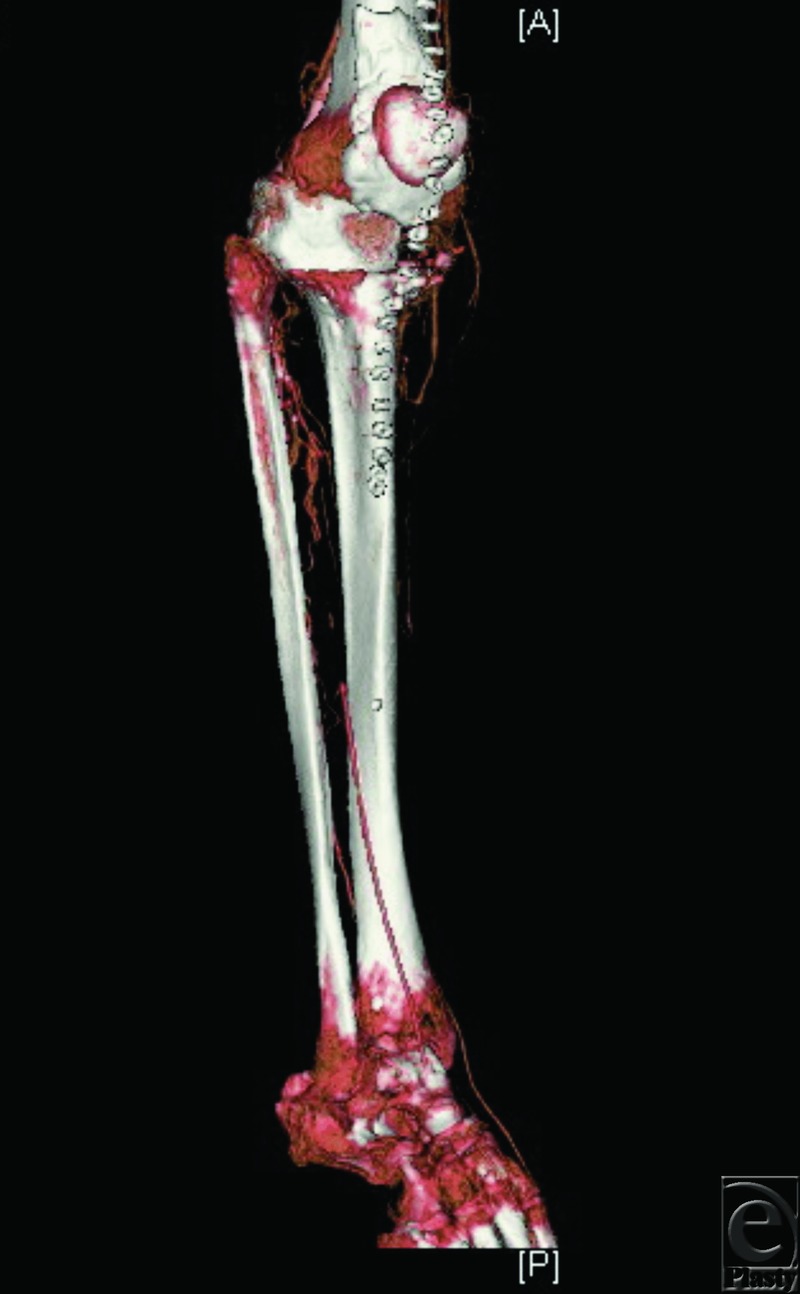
CTA in reconstruction of the lower limb. CTA of a traumatized right lower extremity demonstrating severe atherosclerotic disease as well as numerous focal occlusions of the anterior tibial and posterior tibial arteries distal to the trifurcation of the popliteal artery.
